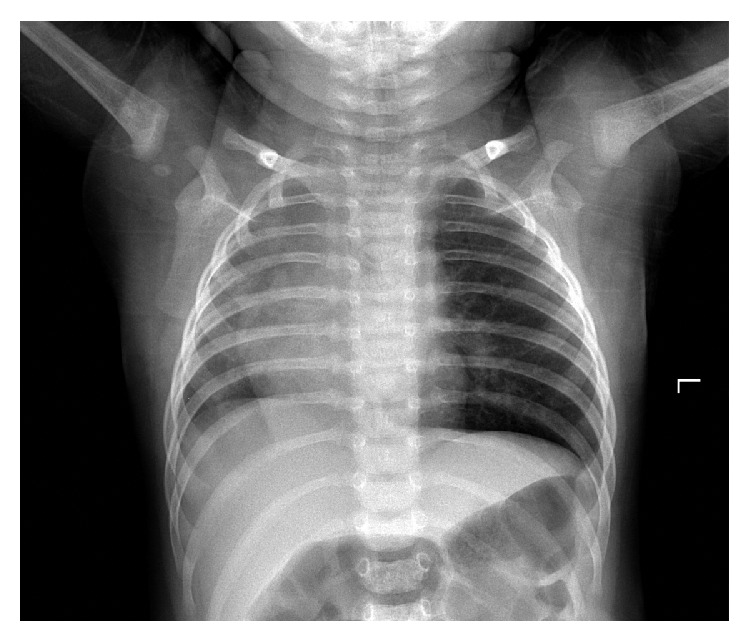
Chest X-ray of a 13-month-old male child showing bronchopneumonia, mediastinum right shift, and emphysema on the left-hand side.
